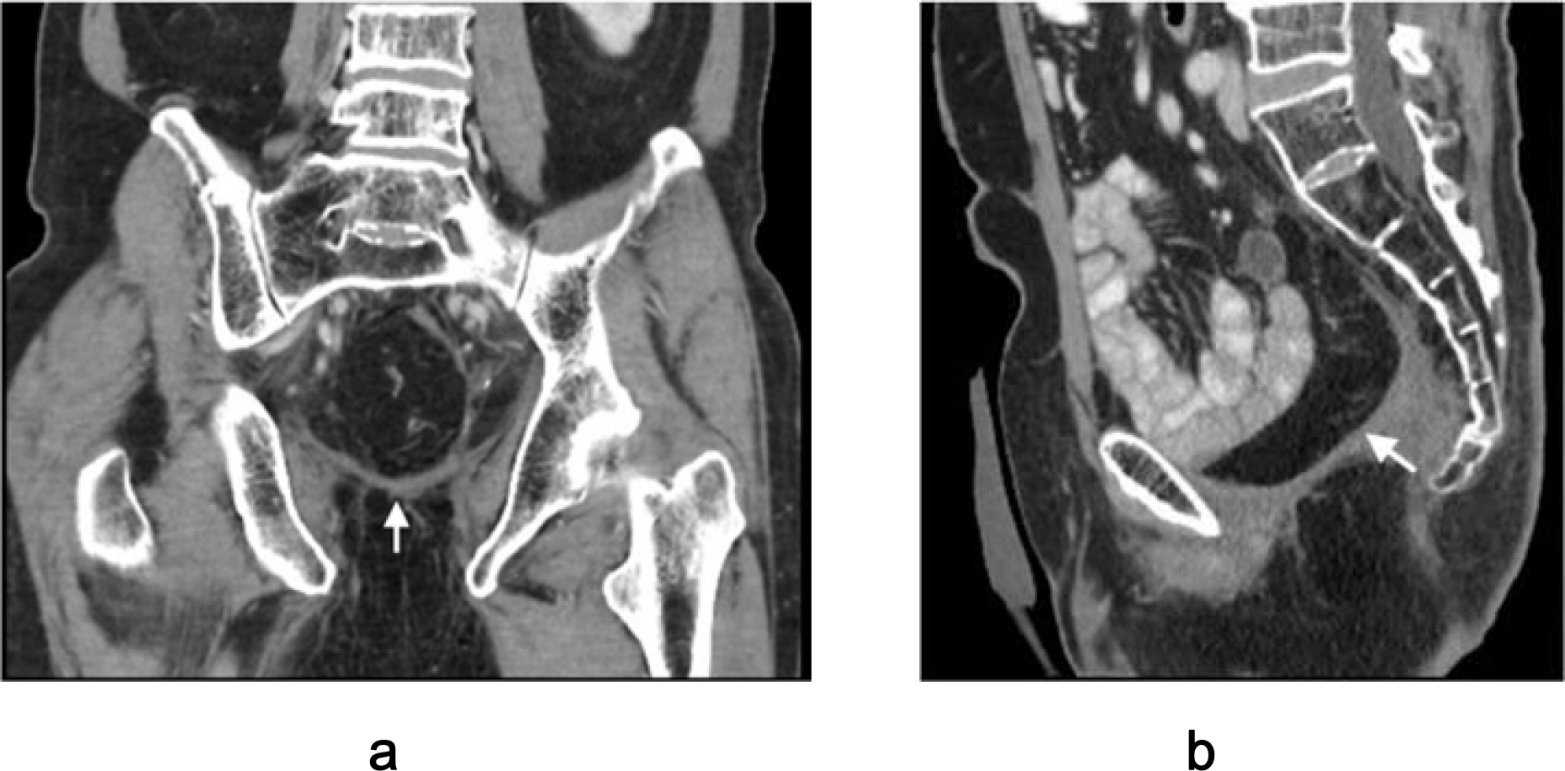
Postoperative CT imaging of mucosa-removed colonic flap. (a) Coronal view; (b) Sagittal view. Arrows indicate optimal alignment of the flap forming a neoperitoneal diaphragm, effectively preventing perineal herniation.
